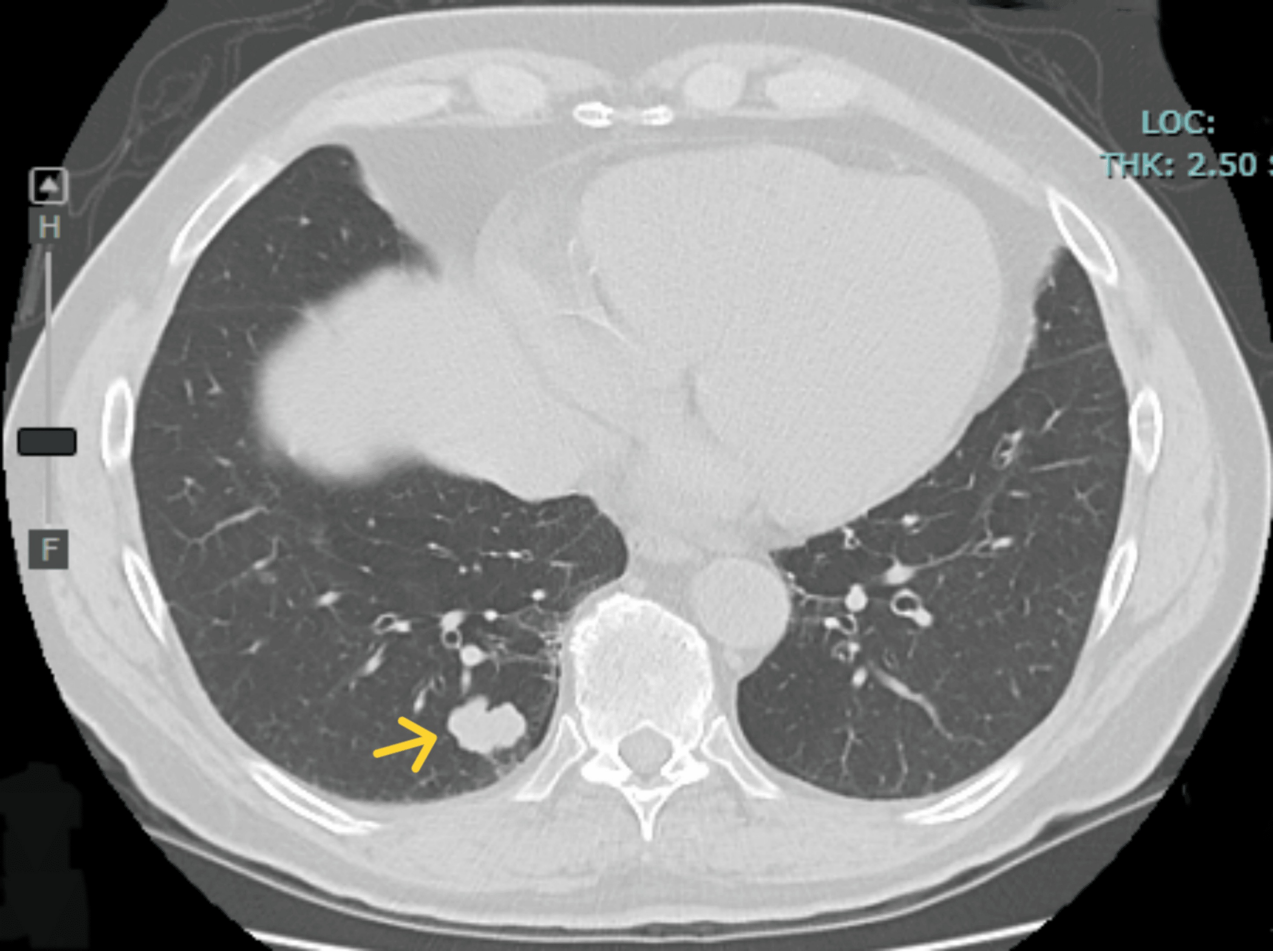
Axial CT of the chest demonstrated a solid pulmonary nodule (yellow arrow) in the right lower lobe CT, computed tomography
